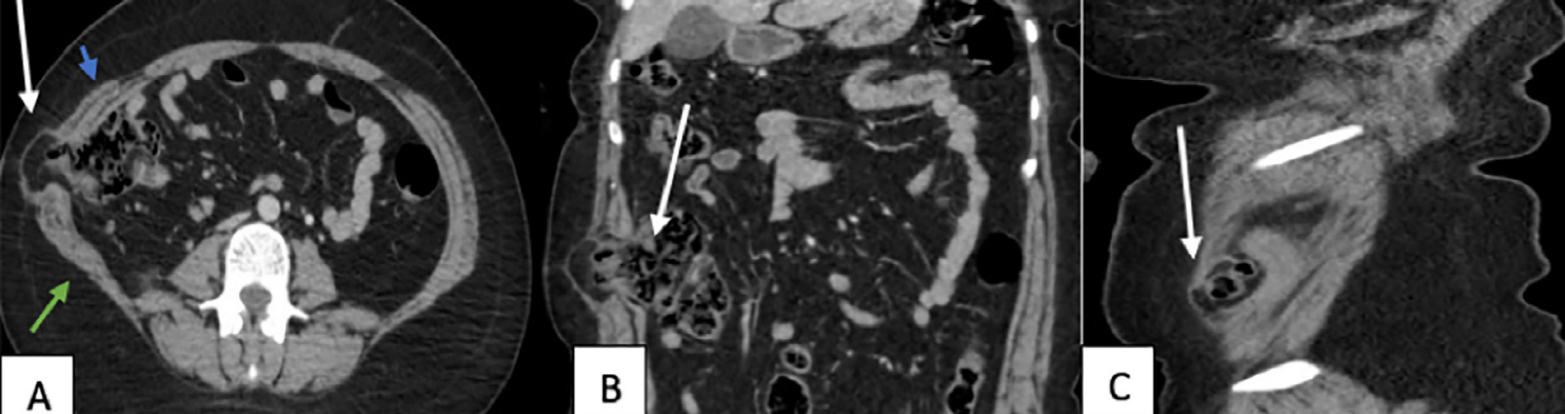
Contrast-enhanced CT scan in the portal phase – axial view (A) with coronal and sagittal reconstructions (B and C). A parietal defect (white arrow) is seen between the external oblique muscle anteriorly (blue arrow) and the latissimus dorsi posteriorly (green arrow) at the level of the inferior lumbar triangle, containing omental and digestive structures without signs of distress.
